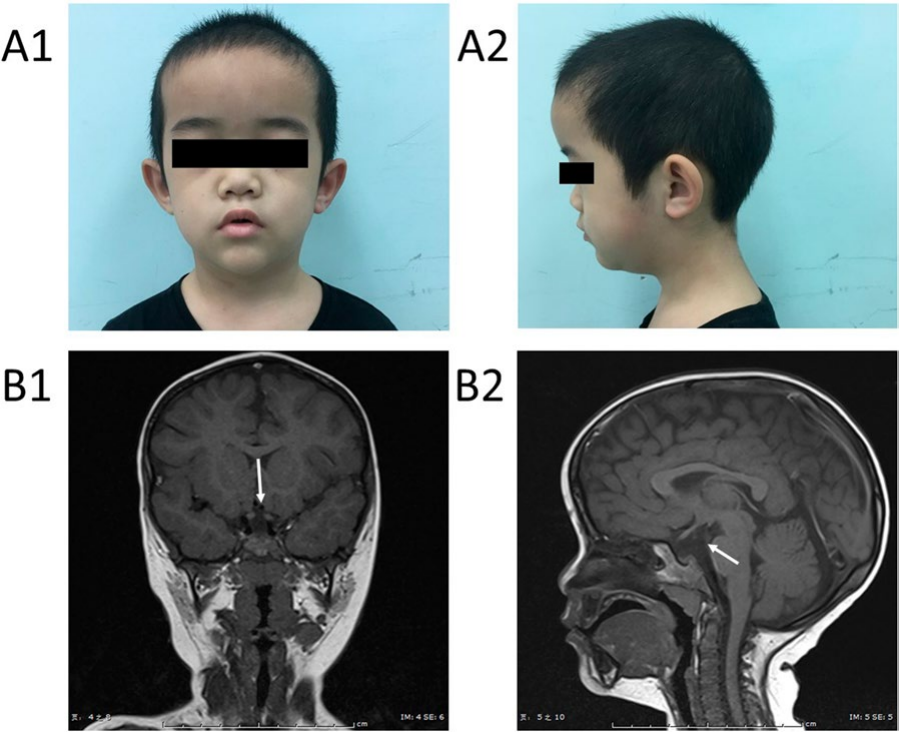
Physical examination results for the 8 -year- old patient. A1, A2 Facial appearance of the patient: slightly larger ears, low ear positions, external rotation, protrusion of ear lobes, thin lips, wide nose bridge, and low forehead hairline. B1, B2 Pituitary MRI of the patient revealed pituitary dysplasia
